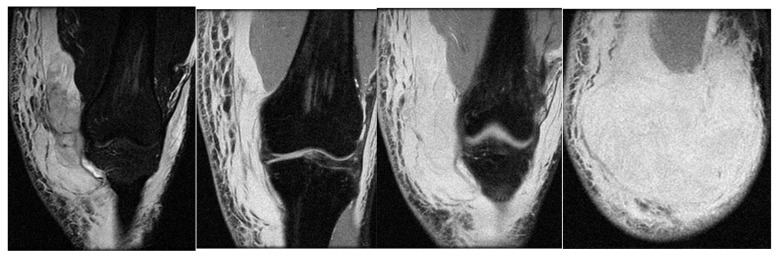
Knee MRI: coronal stir acquisition.
